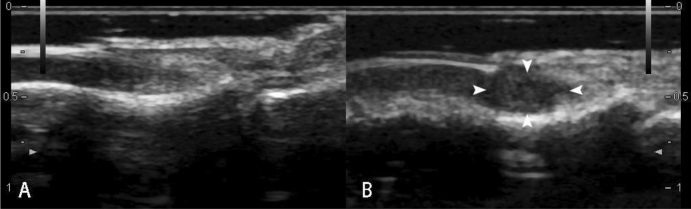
Sonography shows normal nail of the right ring finger (A). Arrow heads point to isoechoic Subungual glomus in the left ring finger (B).
